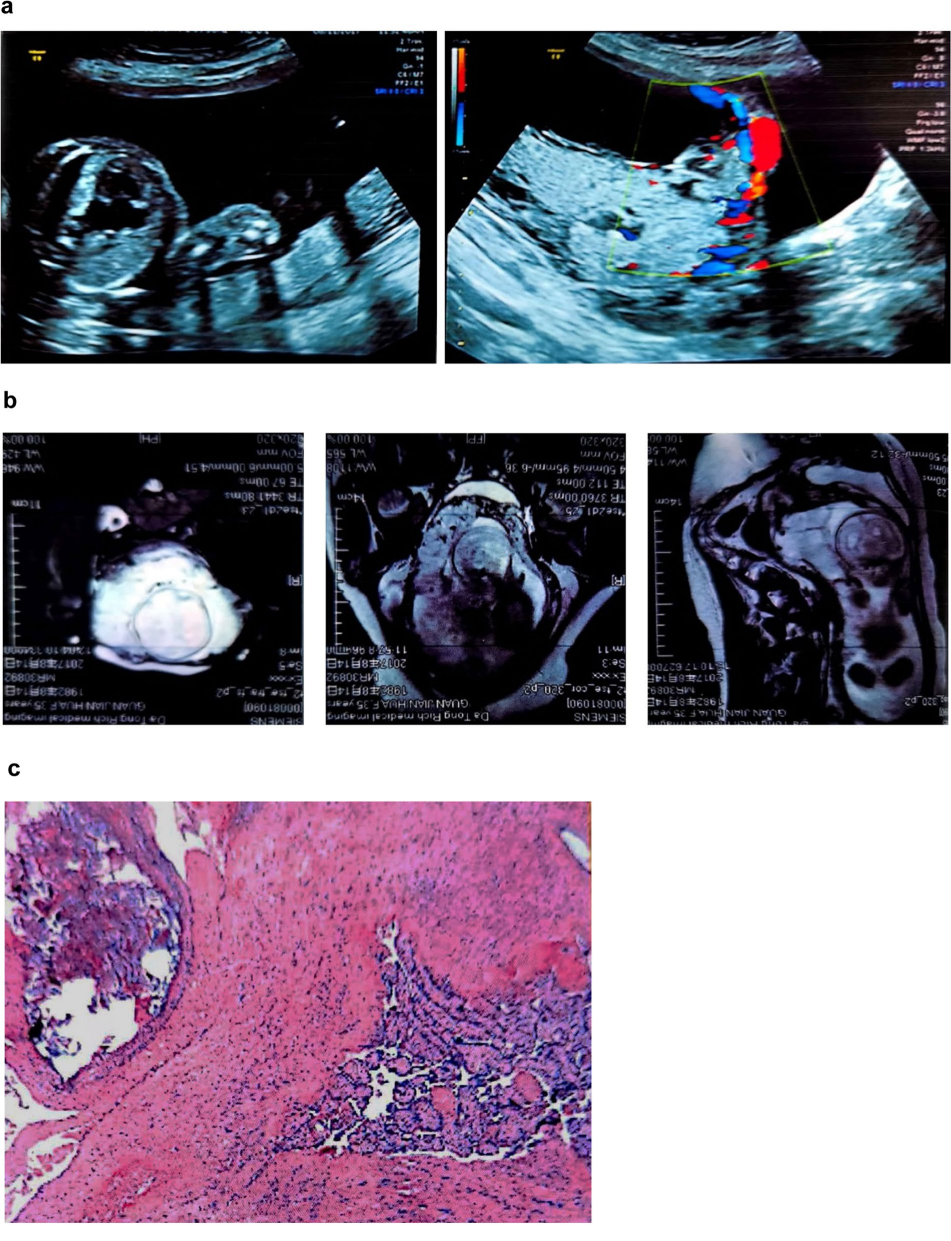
a Ultrasound before termination of pregnancy, b MRI before termination of pregnancy, c Pathology with 100x
